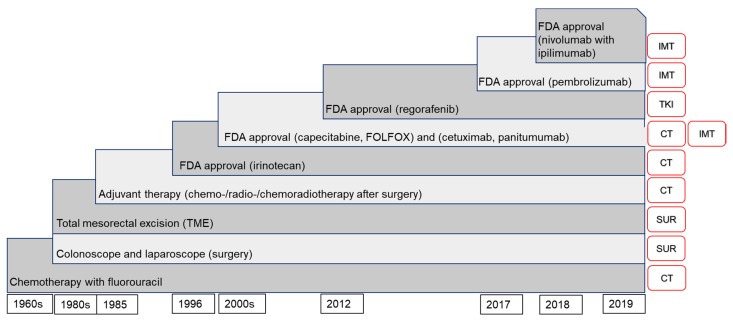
Simplified timeline of colorectal cancer (CRC) treatment options reviewed. The timeline is not constructed according to scale. The red boxes on the right represent the type of treatment, where CT = chemotherapy, SUR = surgery, IMT = immunotherapy, TKI = tyrosine kinase inhibitor.
